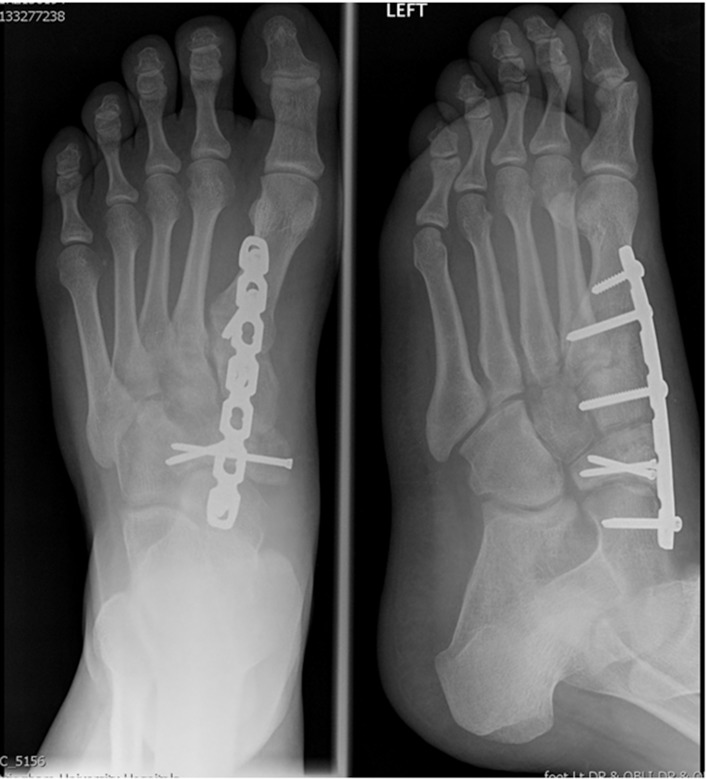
Radiograph following open reduction internal fixation with medial column locking plate
